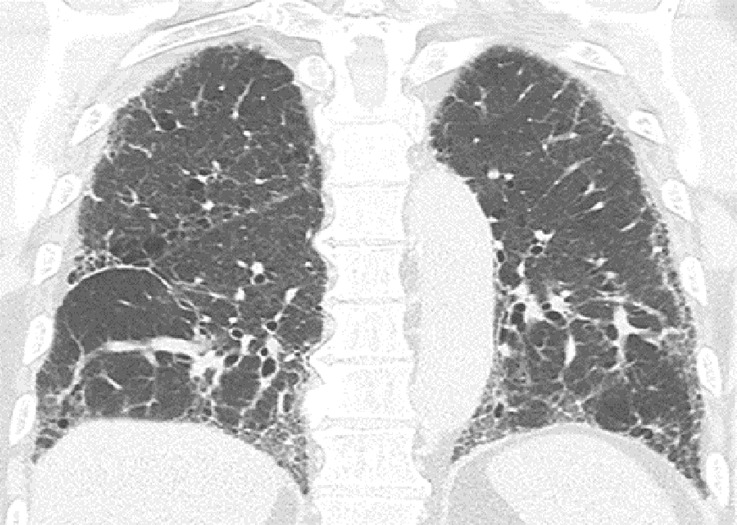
Usual interstitial pneumonia pattern. (a–d) Axial inspiratory CT images demonstrate peripheral and basilar-predominant reticular abnormality, with associated traction bronchiectasis and honeycombing. This is a usual interstitial pneumonia pattern based on Fleischner and American Thoracic Society guidelines. (e) Coronal inspiratory CT scan confirms the lower lung–predominant distribution of disease in the craniocaudal plane.
